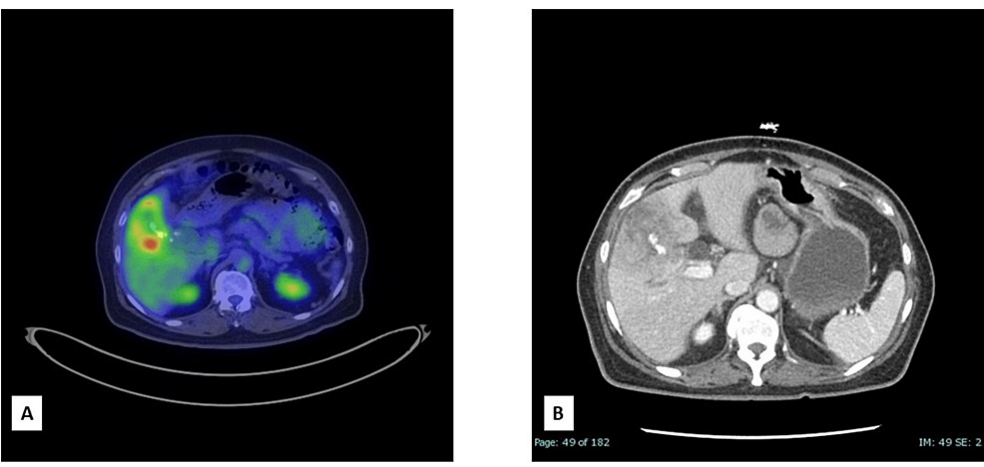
PET-CT and axial contrast-enhanced image of the patient. (A) Axial image from a fluorodeoxyglucose (FDG) PET-CT examination shows a 6.8 x 2.9 cm (AP x transverse) ill-defined low attenuation mass in the inferior liver with internal calcifications and effacement of the gallbladder wall. This mass demonstrates increased FDG activity with a standardized uptake value (SUV) max of 9. (B) An axial contrast-enhanced image of the abdomen redemonstrates irregular gallbladder wall thickening with low attenuation in the surrounding hepatic parenchyma, favored to represent mass invasion. Calcifications within the abnormal gallbladder may represent gallstones. Dilatation of the common bile duct and partially visualized right intrahepatic bile duct dilatation are also observed.
